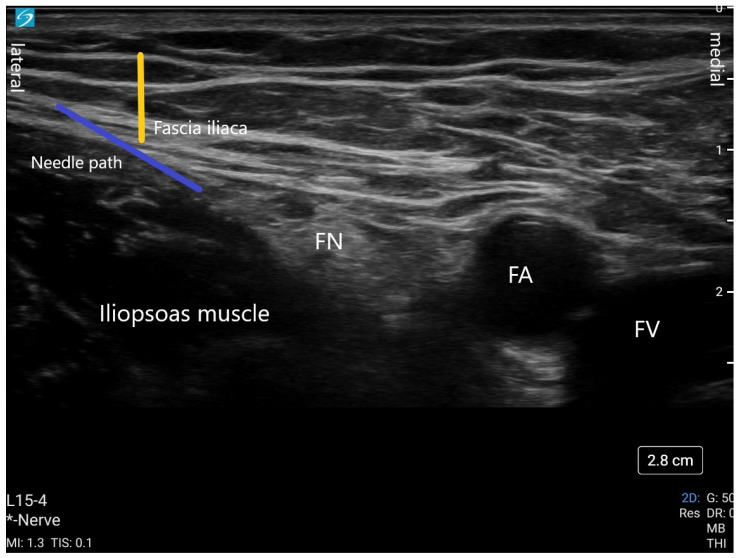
This is an ultrasound image of a femoral nerve block. The yellow line indicates the fascia iliaca plane. The blue line represents the needle trajectory. Local anesthetic is deposited below the nerve and above the nerve. FN (femoral nerve); FA (femoral artery); FV (femoral vein); * Nerve (ultrasound setting is in Nerve mode).
